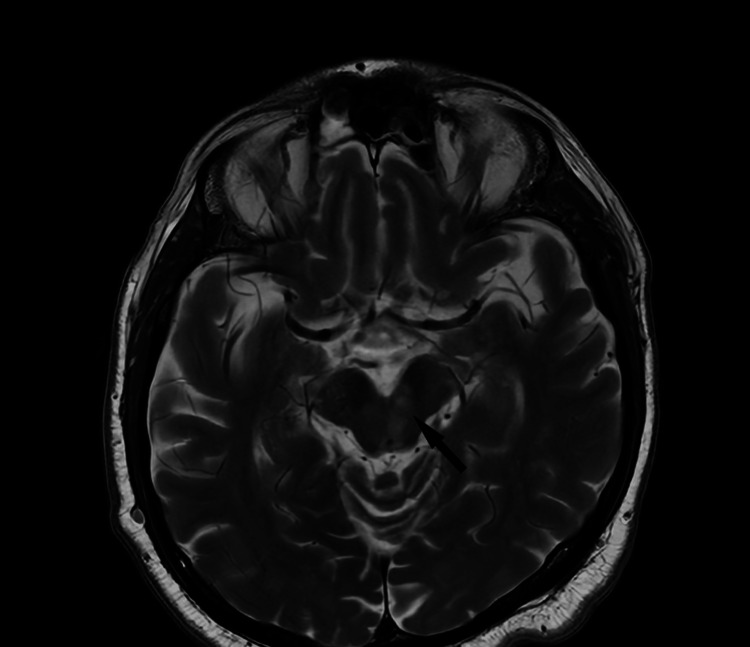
Magnetic resonance tomography of the brain T2-weighted axial scan of the brain: Arrow shows small ischemic lesion in the midbrain
